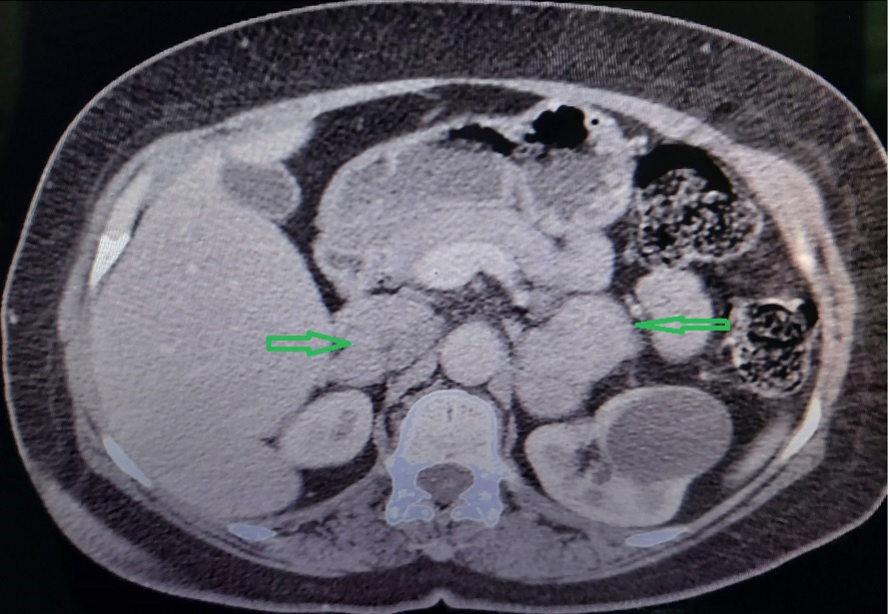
Abdominal computed tomography image showing the bilateral adrenal masses (arrows).
